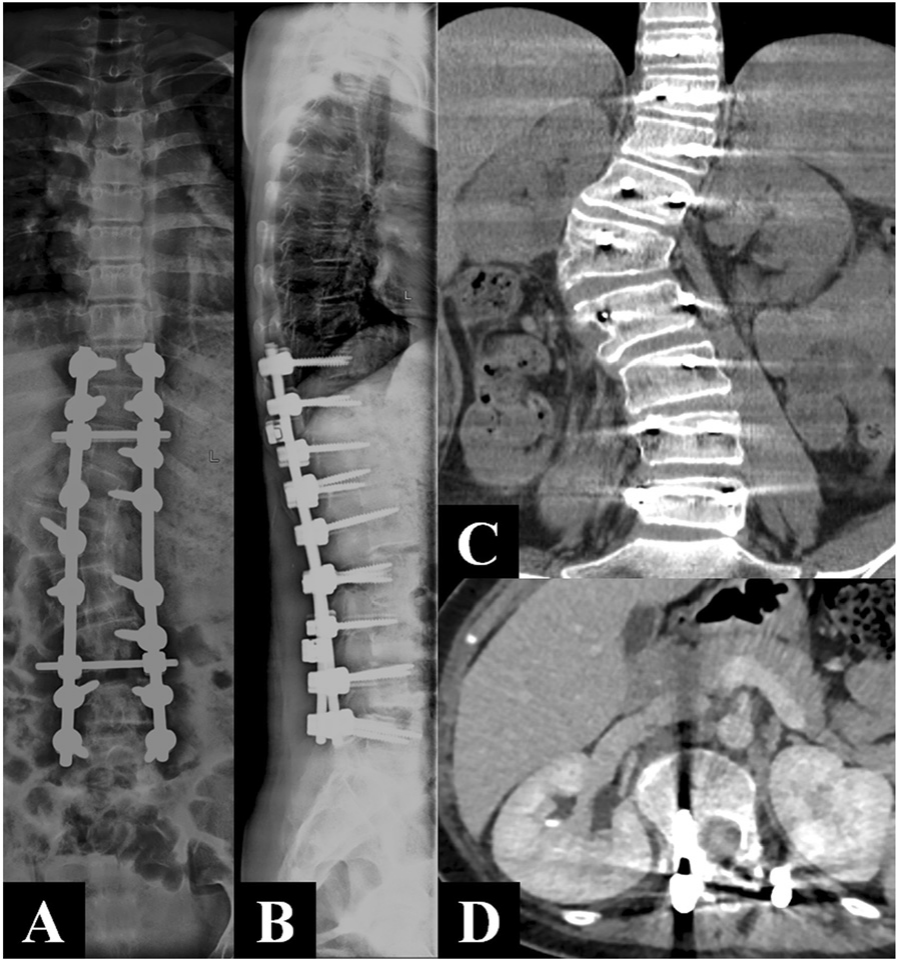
a, b The instrument which is used for internal fixation formed an image in the vertebral body and paravertebral region. The lower segment of the thoracic and lumbar spine existed a right tumefied thoracolumbar curve surrounding the first lumbar body with a Cobb angle of 15.3° and Ferguson angle of 44.3°. c, d CT coronary multi-plane reorganization and axial enhanced scan showed the right paravertebral mass had disappeared, and the kidney was lower compared with that before operation. The right kidney vessels are shown clearly
